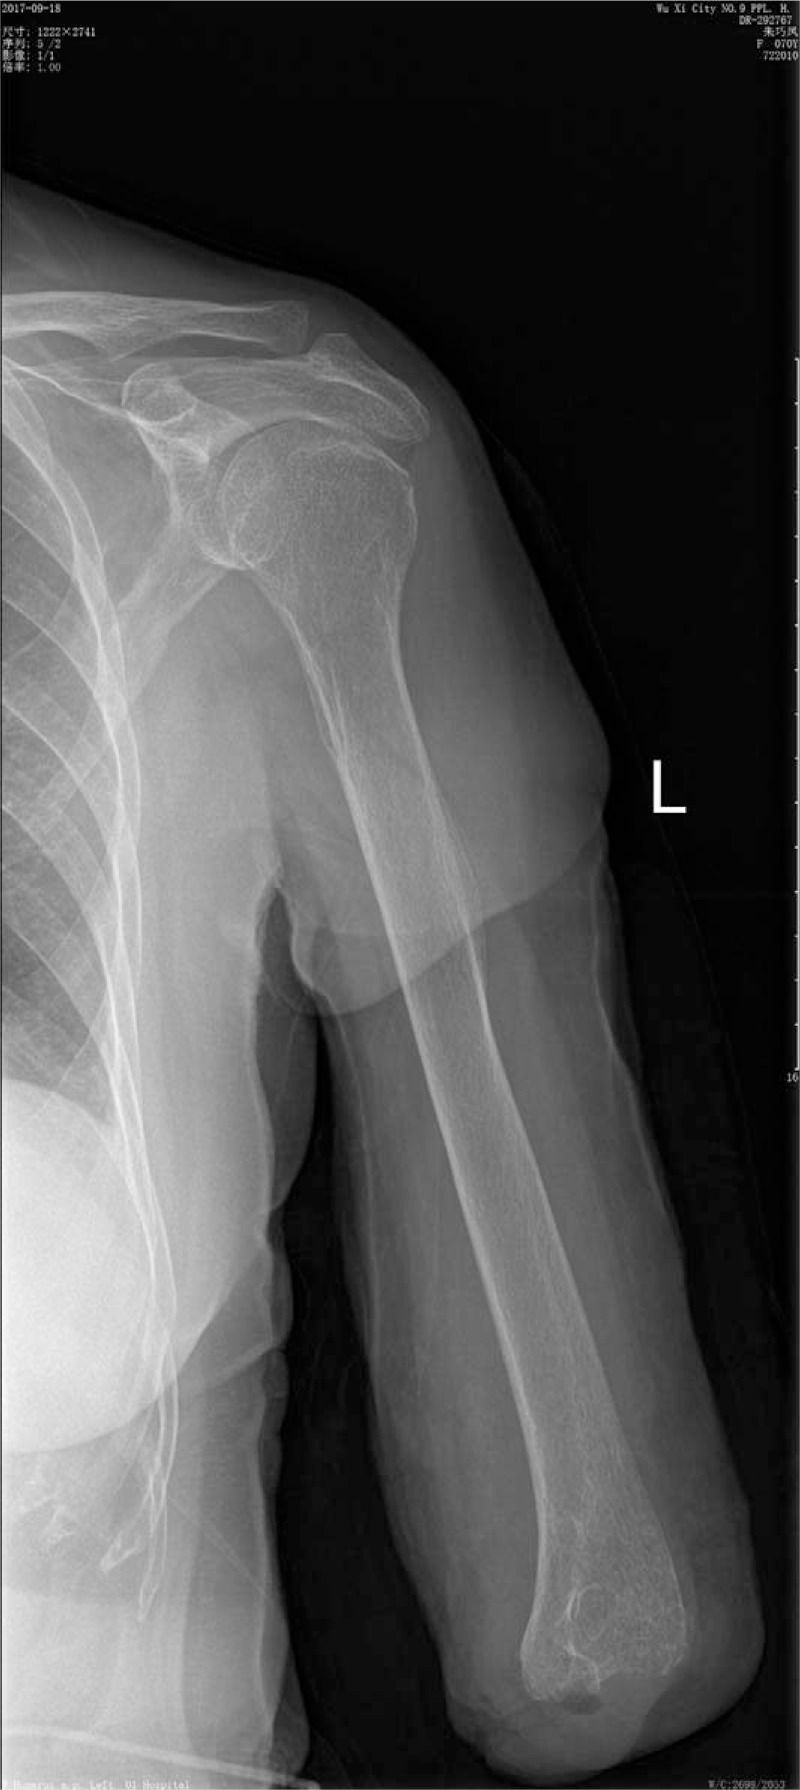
No osteolysis of humerus in x-ray.
